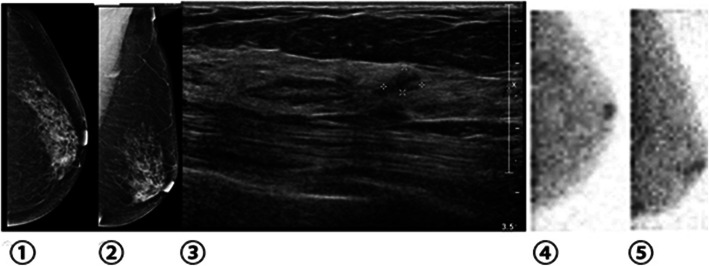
Breast tissue images from a woman. (①②)Mammography (LCC, LMLO) demonstrated a cluster of amorphous micro-calcifications in the upper outer quadrant area, BI-RADS 4B; ③Ultrasound demonstrated a hypoechoic lesion of the left breast, 0.6 cm*0.4 cm, BI-RADS 4A; ④⑤BSGI was negative and the tissue was shown to be normal; Pathology demonstrated this lesion to be a typical ductal hyperplasia
